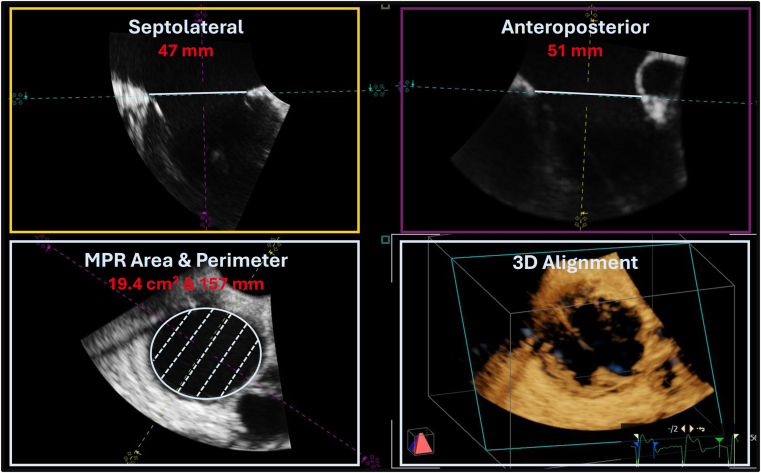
3D TEE multiplanar reconstruction for tricuspid annular dimension assessment. Representative example of preprocedural 3D TEE measurement of TA dimensions using multiplanar reconstruction. Orthogonal long- and short-axis planes were aligned along the TA to enable accurate tracing of its area, perimeter, septolateral, and anteroposterior diameters in early diastole. Abbreviations: 3D, 3-dimensional; MPR, multiplanar reconstruction; TA, tricuspid annular; TEE, transesophageal echocardiography.
